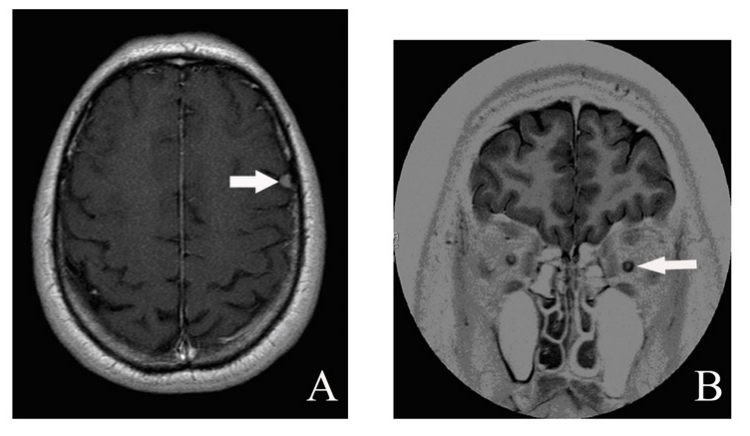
MRI scan for patient I-2. A) Left frontal meningioma measuring 4 mm in diameter. B) Atrophy of the two optic nerves in their intraorbital portions (before the intracanal portion) with dilatation of the sheaths.
